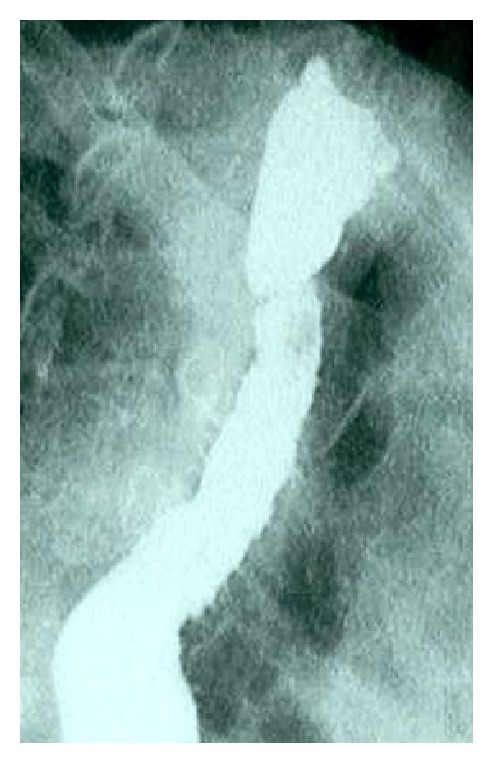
Esophageal barium contrast radiography with a proximal esophageal stricture and small areas of contrast enhancement parallel to the esophageal wall, compatible with esophageal intramural pseudodiverticulosis.
